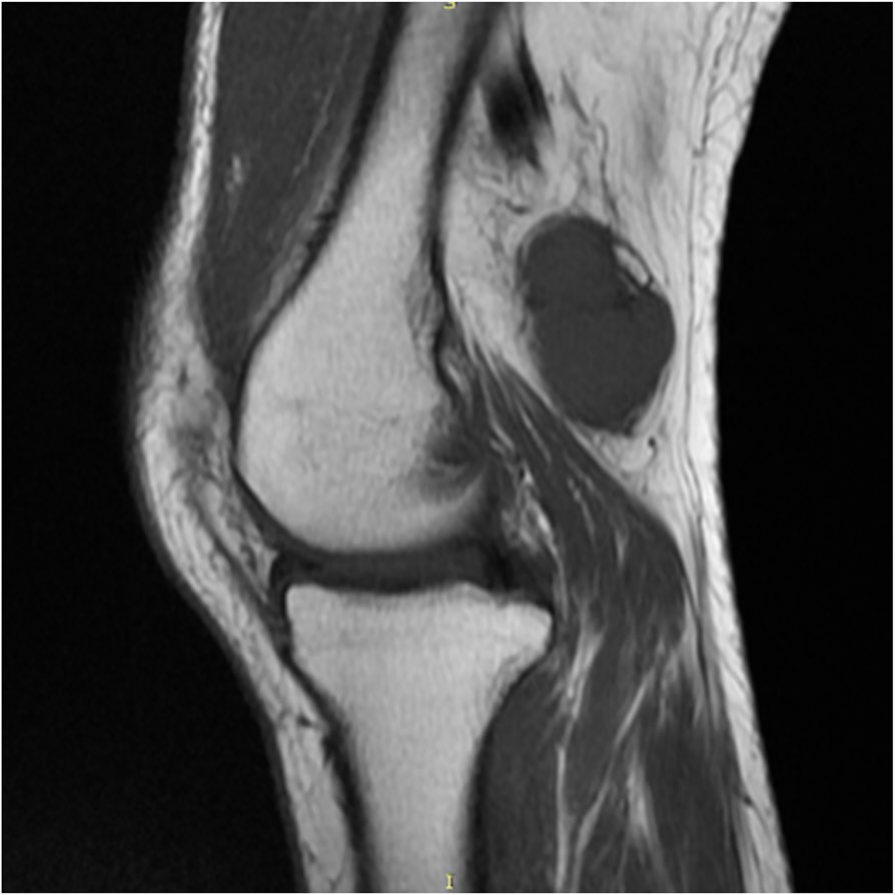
MRI T1 weighted image (sagittal view) showed a hypointense to isointense mass in the left popliteal fossa
